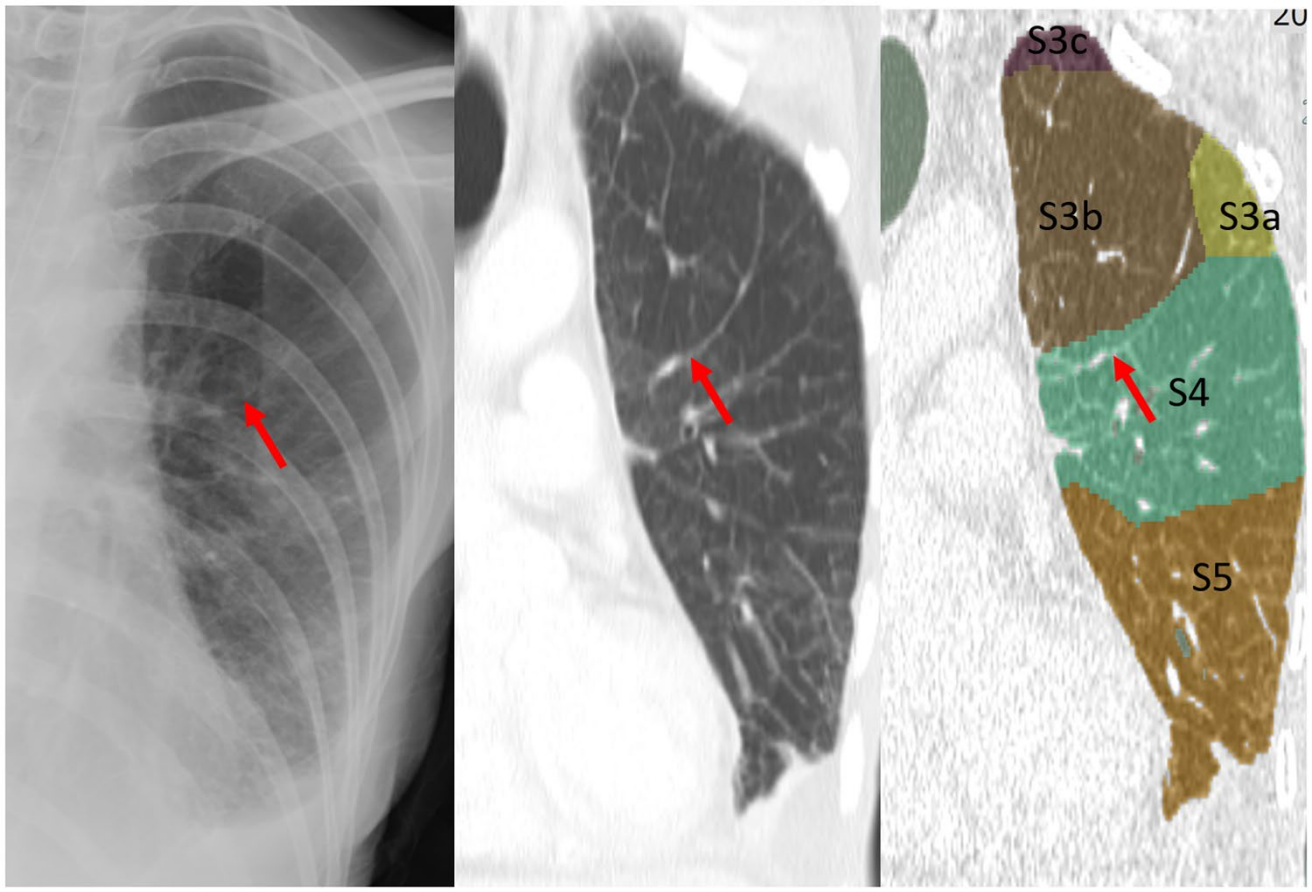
Case 3. Line 5. 51-year-old woman with gastric cancer. Chest X-ray shows the Kerley A-line in the left lung. The line corresponds to the thickened septal line on CT and matched with the segmented lines between S3b and S4 by 3D segmentation analysis
